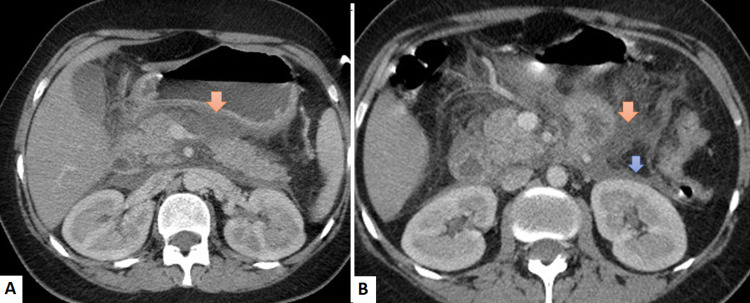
Contrast-enhanced computed tomography images revealing acute pancreatitis A: Diffusely edematous pancreas with peripancreatic fluid collection (orange arrow) B: Perinephric fascia thickening (blue arrow)
